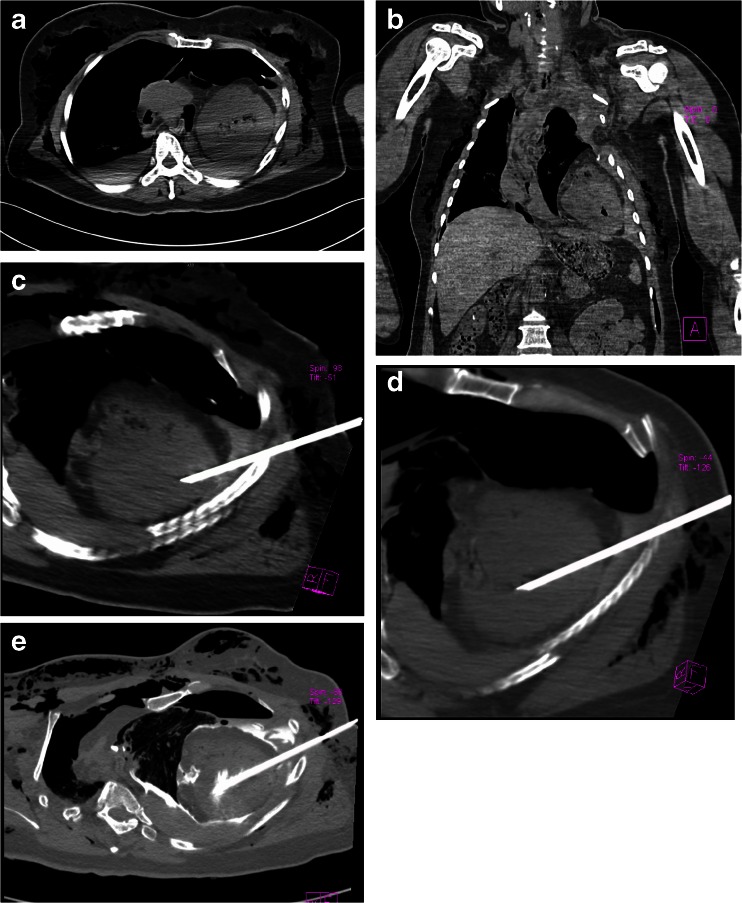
PMCTA procedure. a, b PMCT revealed fallen of the heart into the left costophrenic angle and pleural effusion. c, d A percutaneous puncture into the left ventricle through the intercostal area between the left fifth and sixth rib was conducted under CT guidance. e Administration of the contrast media
